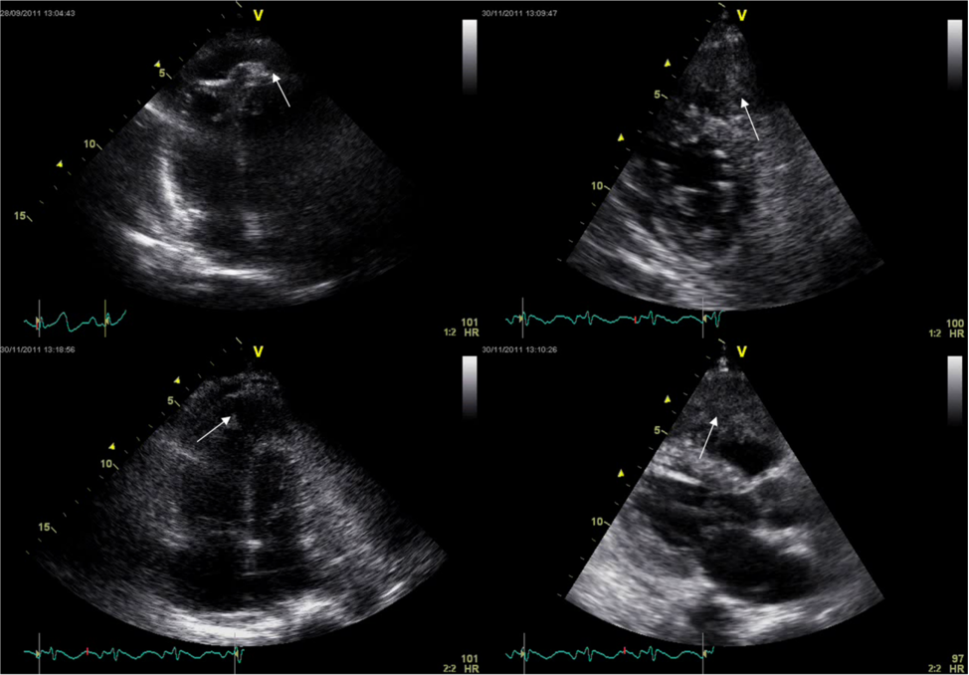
Echocardiographic evaluation. Four-chamber 2-dimensional transthoracic echocardiogram shows a large, irregular metastatic mass (2.3×1.1 cm), which infiltrated pericardium and myocardium (predominantly anteroapical and lateral walls of the left ventricle) with intracavitary propagation (arrows).
